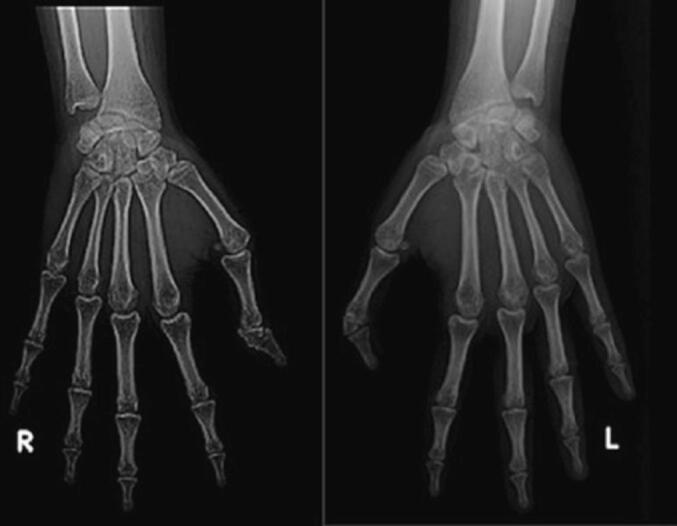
X-ray of both thumbs at pre-operative condition.
